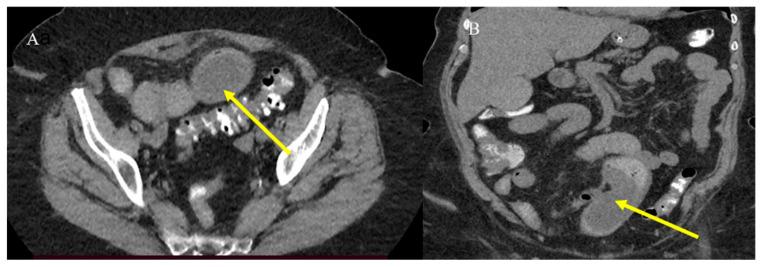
The CT scan shows integration with GGF in the previous case of the 72-year-old woman with sub-occlusive symptoms and an intestinal polyp. The intestinal polyp is highly visible in images (A,B) after the small bowel lumen opacification by GGF as an ipodense mass (yellow arrow), and the sub-occlusive symptoms were resolved as the GGF progressed in the sigma and rectum.
